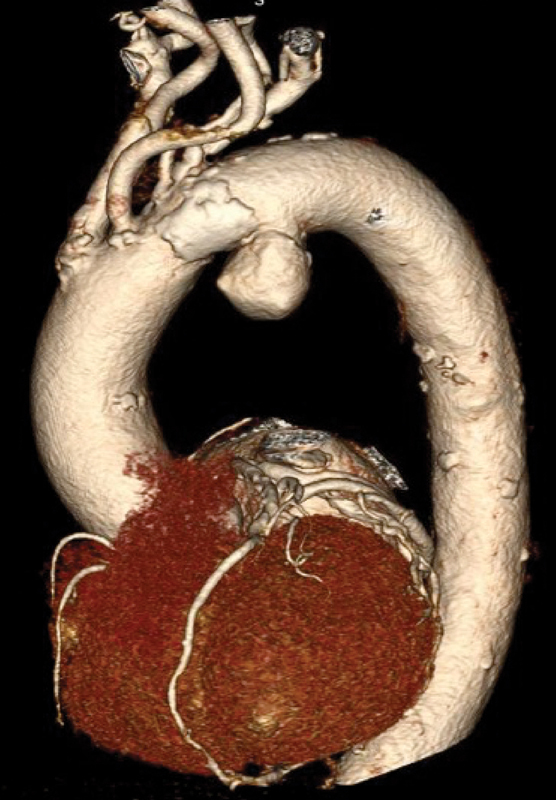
Three-dimensional reconstruction computed tomography angiography of the aortic arch showing a 5.5 cm saccular arch aneurysm. Note the location of the aneurysm—where the ligamentum arteriosum attaches to the aorta. The left vertebral artery (LVA) comes off the aorta just before the left subclavian artery. LVA was not revascularized in this case as the patient was found to have a dominant right vertebral artery and patent basilar artery.
